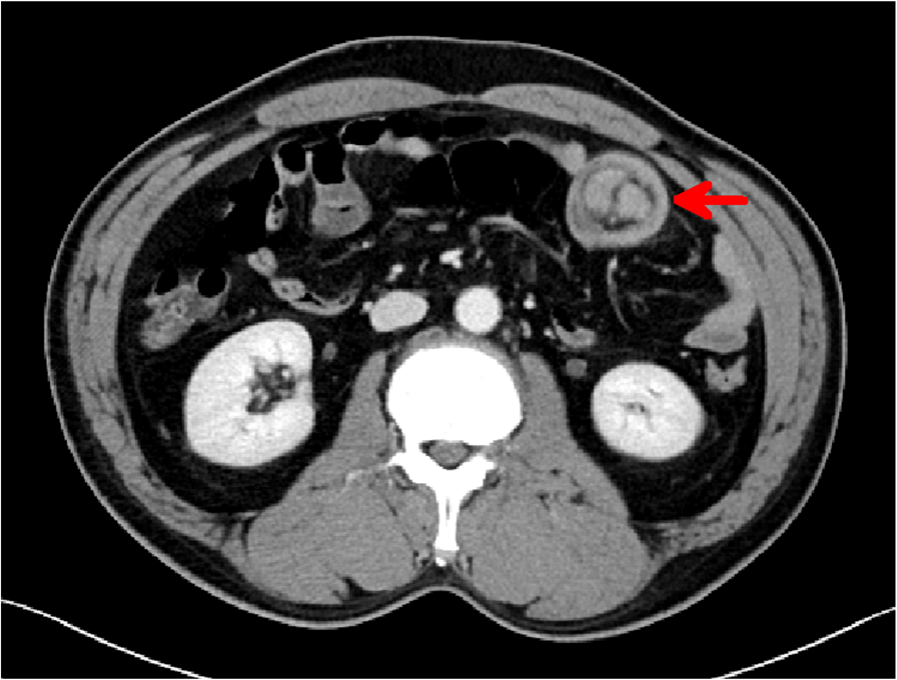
CT scan showing a target sign at the jejunum
